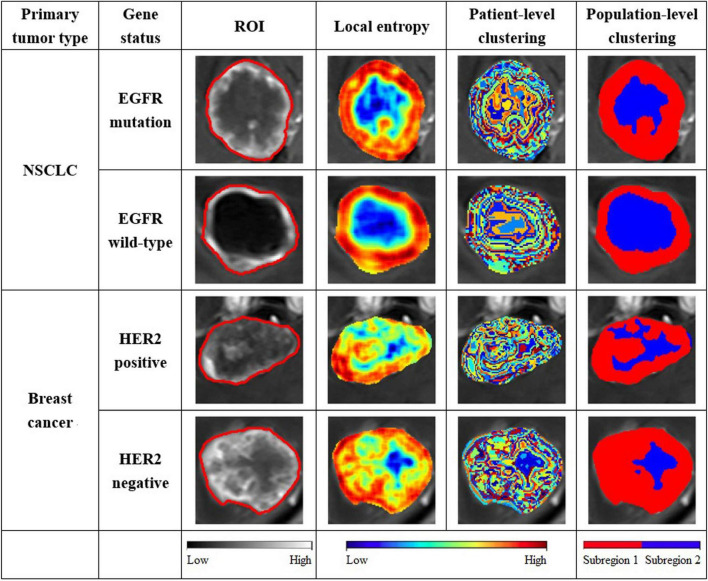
Intratumoral partitions in the MRI images of patients with brain metastasis. The third column represents ROIs in the MRI image. The fourth column represents local entropy maps within the tumor. The fifth column represents the results of patient-level clustering. The sixth column represents the results of population-level clustering.
